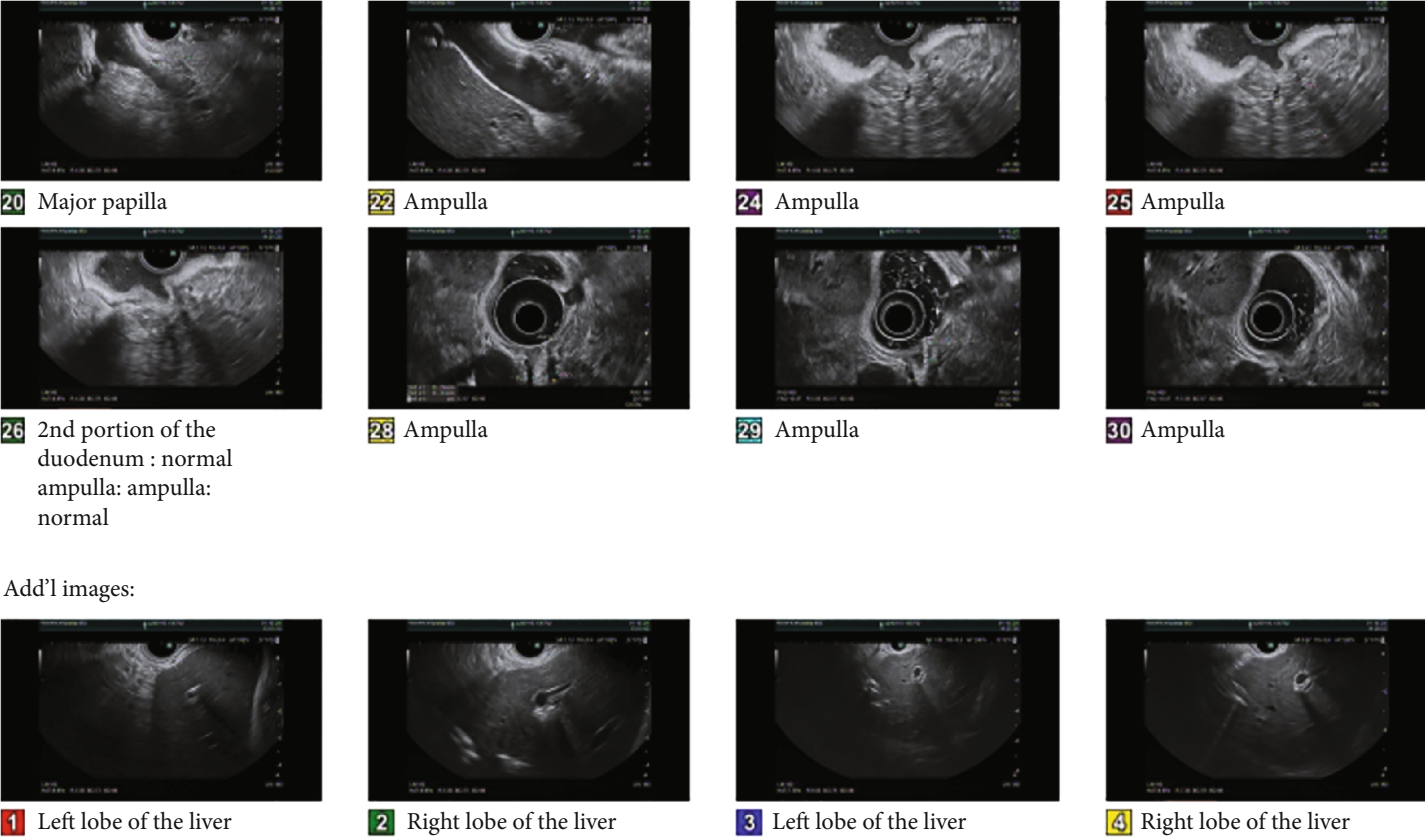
EUS showing no significant endosonographic abnormalities in the ampulla. A prior sphincterotomy site was noted. No pathological lymphadenopathy, masses, or wall thickening was identified.
